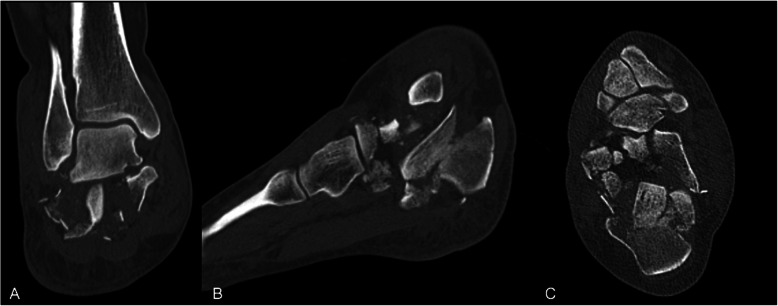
Case 2 Preoperative CT Coronal (A) sagittal (B) and axial (C) views representing a Sanders IV fracture type pattern
